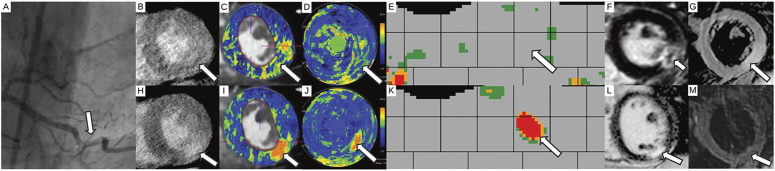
Myocardial injury with microvascular obstruction (MVO) in spontaneous coronary artery dissection in a 45-year-old female (*patient 13*) with (A) dissection of the distal right coronary artery in invasive coronary angiography (arrow). B, Late enhancement CT performed 6 days after symptom onset revealed focal inferolateral hypodensity (arrow) with surrounding late enhancement. Areas of elevated ECV surrounding the central hypodensity are visible in (C) overlay images and on (D) polar maps, with AHA segmental ECV distribution in a circular display (arrows), but no substantial increases are seen in the corresponding atlas map (E), due to smoothing effects from median filtration suppressing small high-contrast areas such as MVO (arrow). F, Late gadolinium enhancement cardiac MRI 2 days prior to CT showed an inferolateral infarct including MVO and edema on (G) T2-weighted cardiac MRI (arrows). In the acute phase, MVO led to diminished perfusion resulting in reduced late enhancement and extracellular volume elevations at the center of the infarct. Nonetheless, the surrounding pathologic myocardium was identifiable via overlay images and polar maps. H, Follow-up CT 117 days after symptom onset revealed an inferolateral myocardial infarct with ECV elevations in (I) overlay images, on (J) polar and on (K) atlas maps (arrows). L, Late gadolinium enhancement (arrows) shows persistent late gadolinium enhancement in the inferolateral wall and (M) T2-weighted cardiac MRI demonstrated resolution of edema (arrows).
